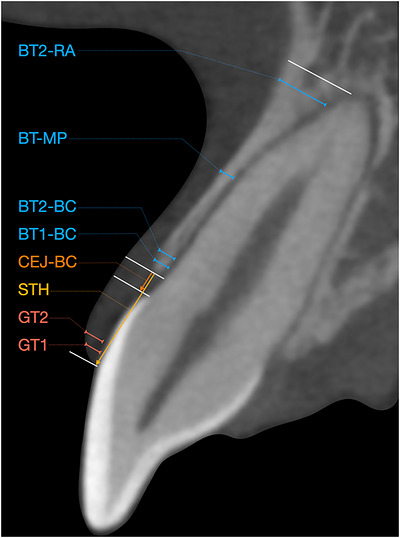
Mid‐sagittal radiographic section demonstrating the methodology applied to make linear measurements of GT1 (gingival thickness at 1 mm apical to the gingival margin); GT2 (gingival thickness at 2 mm apical to the gingival margin); STH (supracrestal tissue height); CEJ‐BC (distance between cementoenamel junction and bone crest); BT1‐BC (buccal bone thickness at 1 mm apical to the buccal crest); BT2‐BC (buccal bone thickness at 2 mm apical to the buccal crest); BT2‐RA (buccal bone thickness at 2 mm coronal from the radiographic apex); and BT‐MP (buccal bone thickness at midway between BT2‐BC and BT2‐RA).
